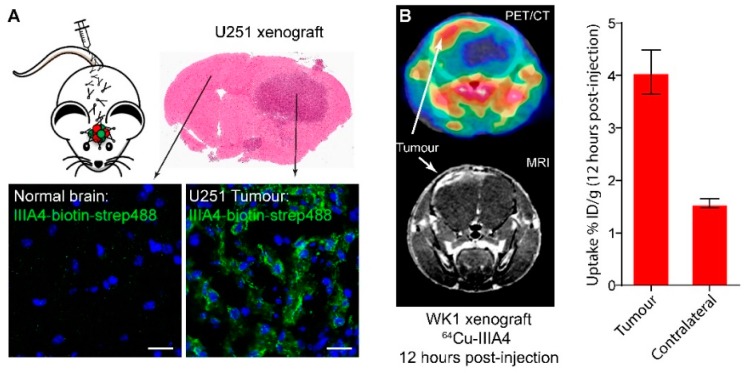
IIIA4 crosses the blood-tumour barrier (BTB) and accumulates specifically in GBM. (A) Tumour uptake of biotin-labelled IIIA4 (shown in green) 2 h post IV injection in a U251 orthotopic tumour xenograft, compared to adjacent normal brain was visualized with streptavidin-Alexa Fluor 488. (B) positron emission tomography/computed tomography (PET/CT) imaging and image quantification of ^64^Cu-labelled IIIA4 uptake into MRI-confirmed tumour tissue in an EphA3^+^ intracranial primary GBM tumour model (WK1). Images were acquired 12 h after IV injection of the labelled antibody. Scale bars represent 50 μm.
